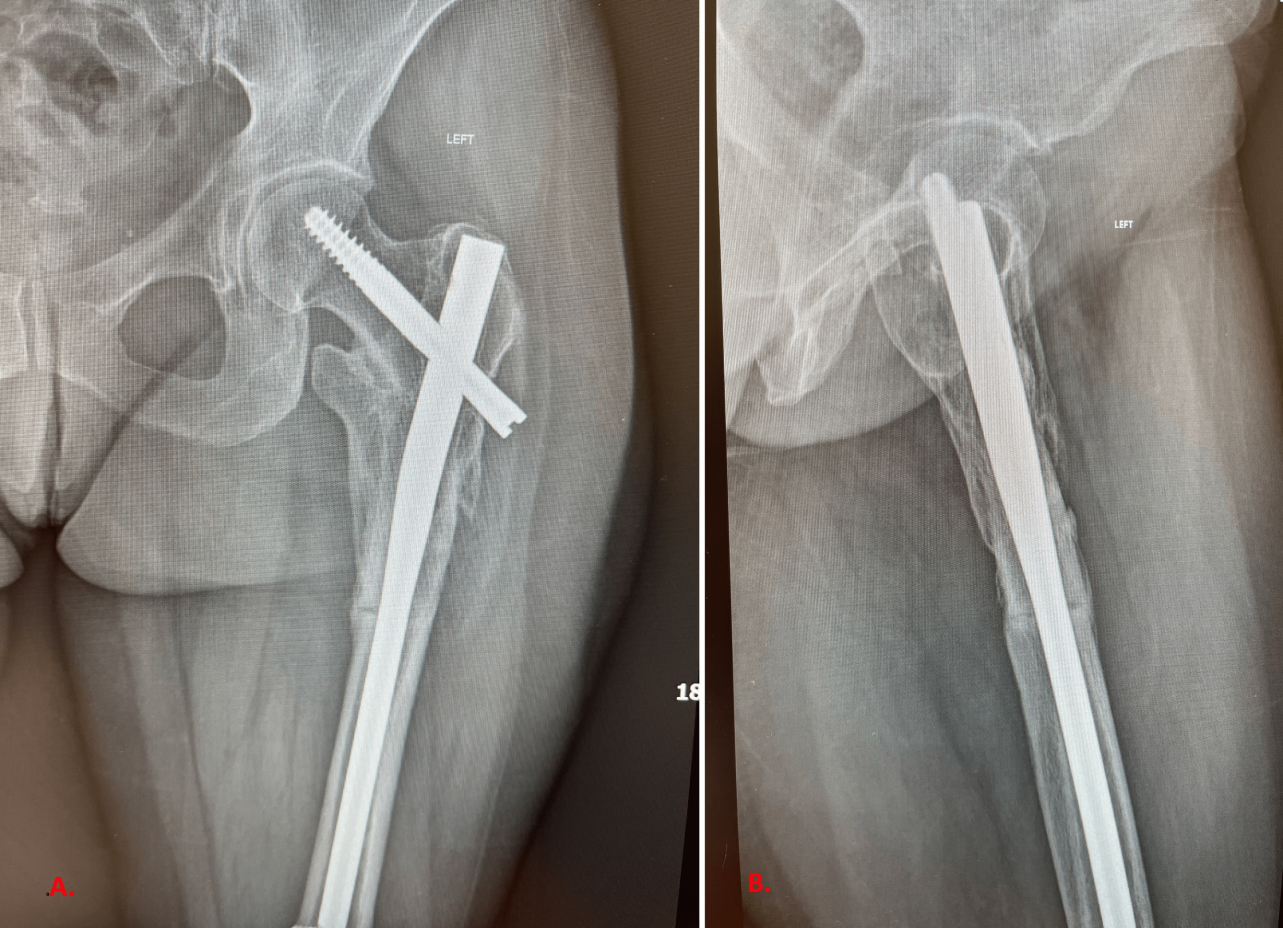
Follow-up radiographs (A, B) Left hip AP and lateral radiographs, respectively, obtained at the four-month follow-up visit.
